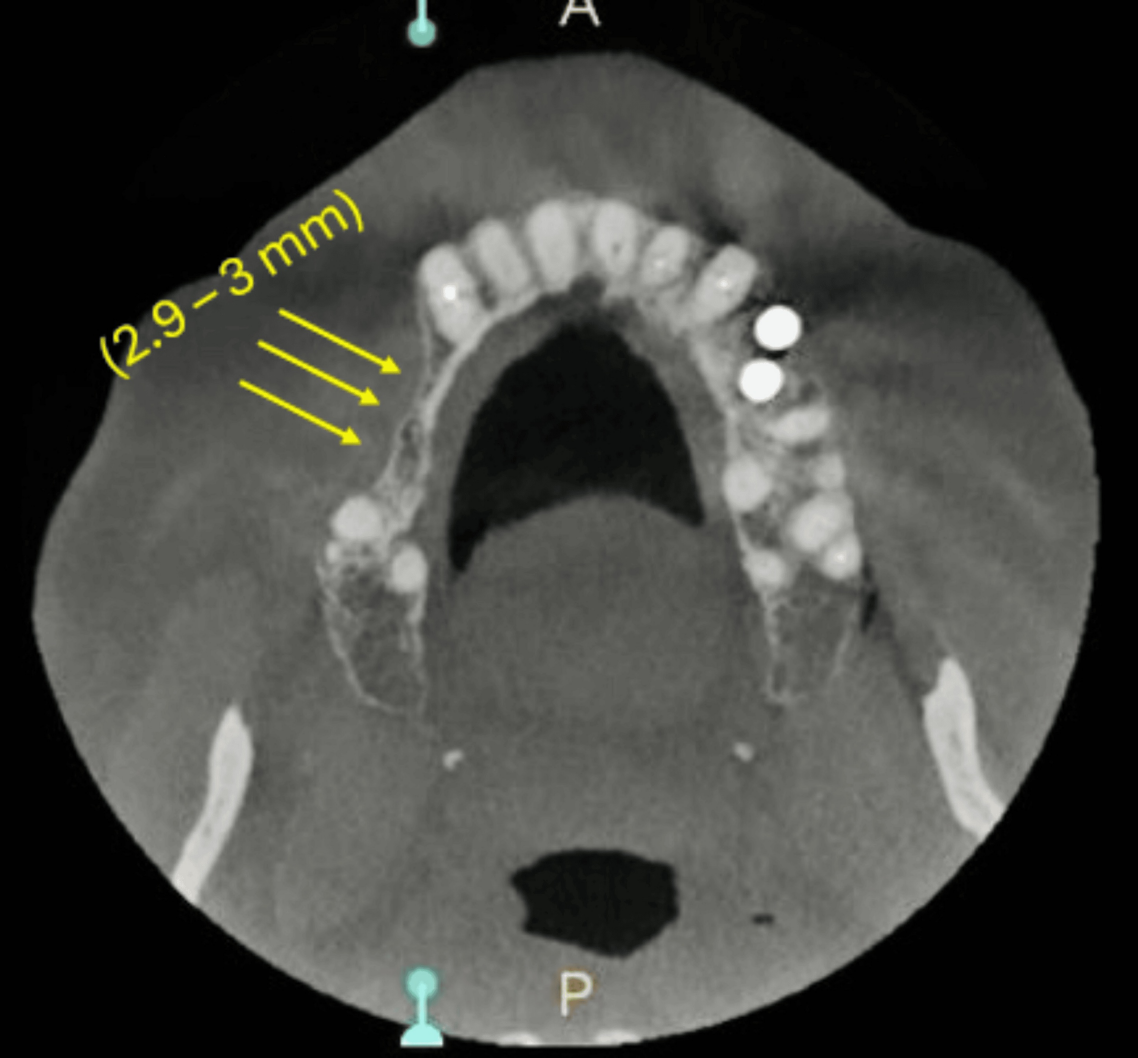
Axial view of CBCT The yellow arrows in the picture indicate the reduced buccolingual width (2.9-3 mm) of the alveolar bone. CBCT, cone beam computed tomography
